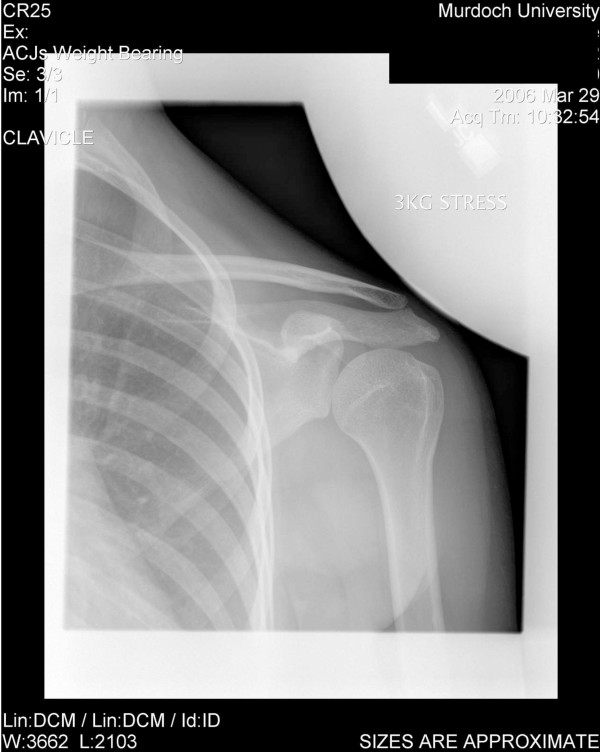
see attached jpeg file named "XRay 3".
